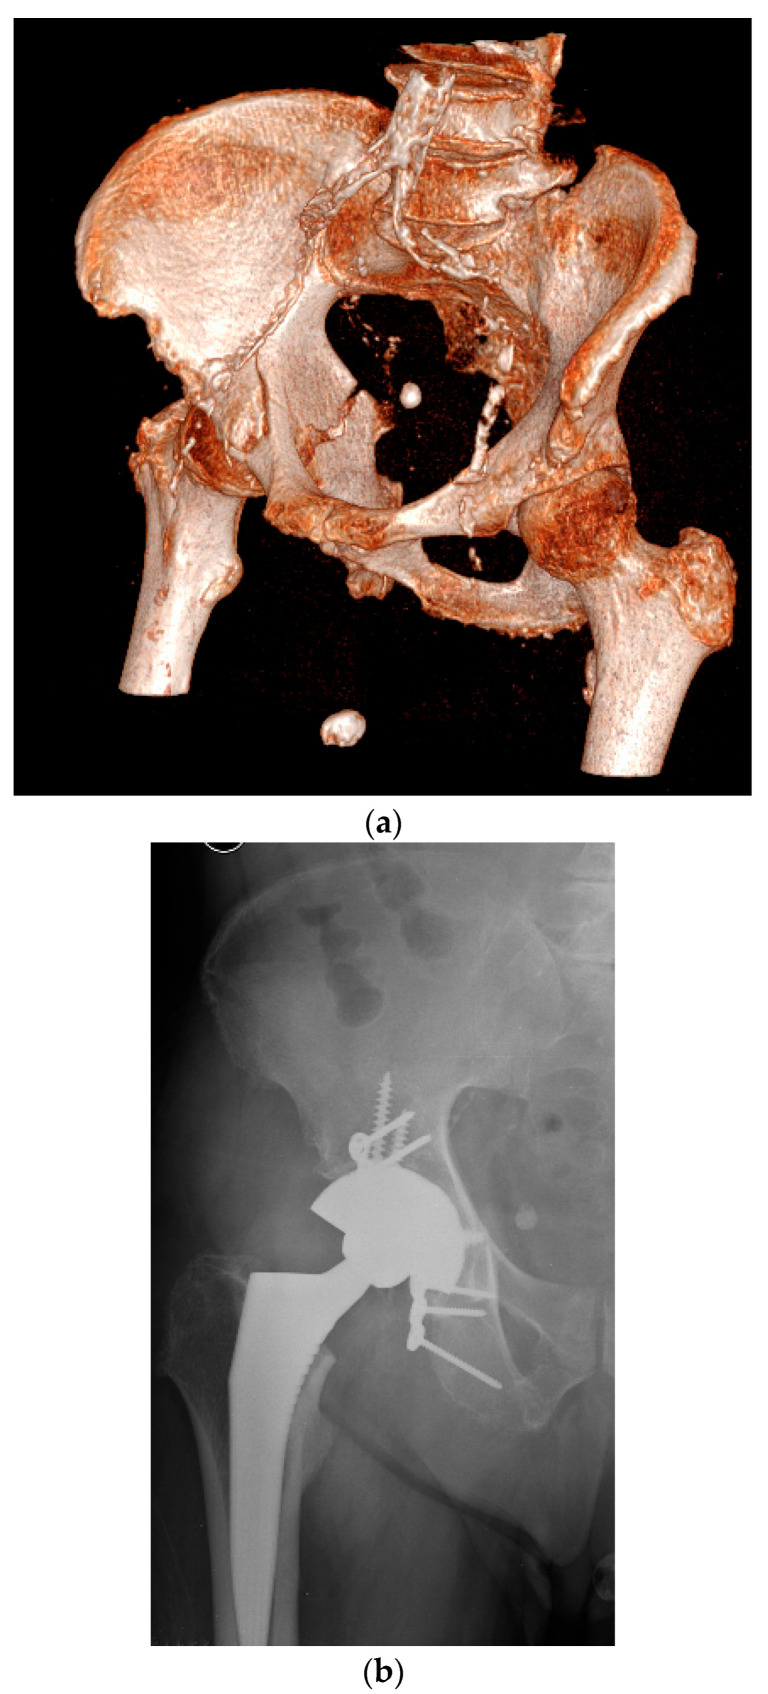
(a) Three-dimensional CT reconstruction. (b) Postoperative radiograph with standard multi-hole acetabular cup and posterior plate fixation.
